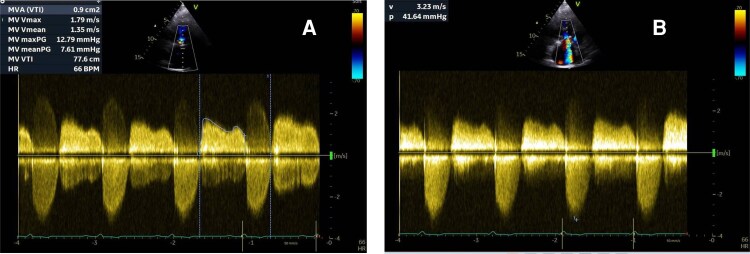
(*A*) CW Doppler apical 4-chamber (A4C) of tricuspid bioprosthesis. (*B*) CW Doppler apical 4-chamber of tricuspid regurgitation peak velocity (*V*_max_).
